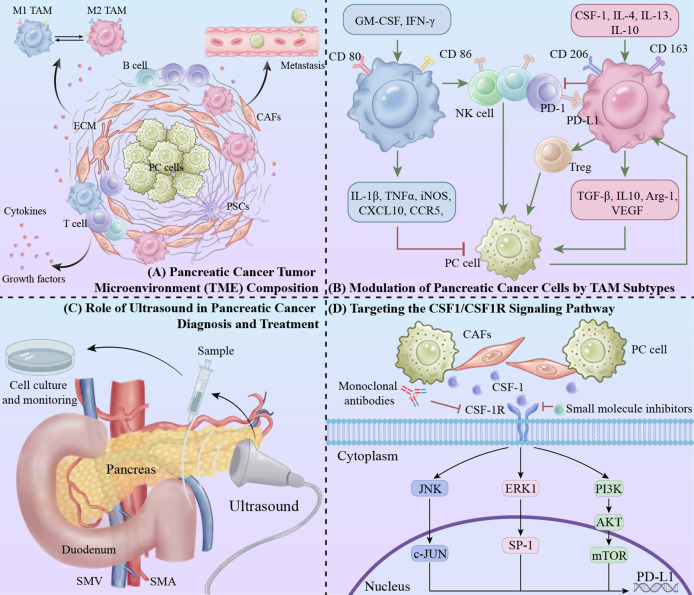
Integrative approaches in pancreatic cancer: targeting tumor microenvironment and macrophage modulation via ultrasound and CSF1/CSF1R pathway inhibition. (A) Pancreatic cancer TME composition. (B) Modulation of pancreatic cancer cells by TAM subtypes: The red “T” symbol indicates inhibitory effects, while the green arrow represents promotive actions within the signaling pathways. (C) Role of ultrasound in pancreatic cancer diagnosis and treatment. (D) Targeting the CSF1/CSF1R signaling pathway.
